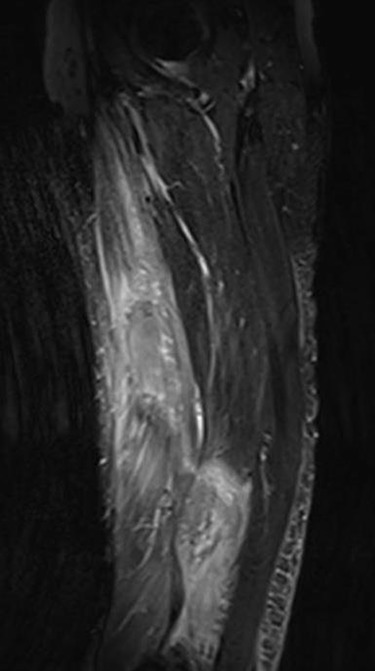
MRI coronal view displaying hematoma and rupture of sartorius muscle.
